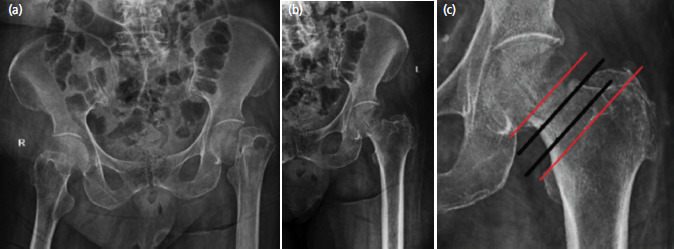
(a, b, c) Radiographs depicting 82-year-old female with Singh index grading 3 who sustained a subcapital fracture.
